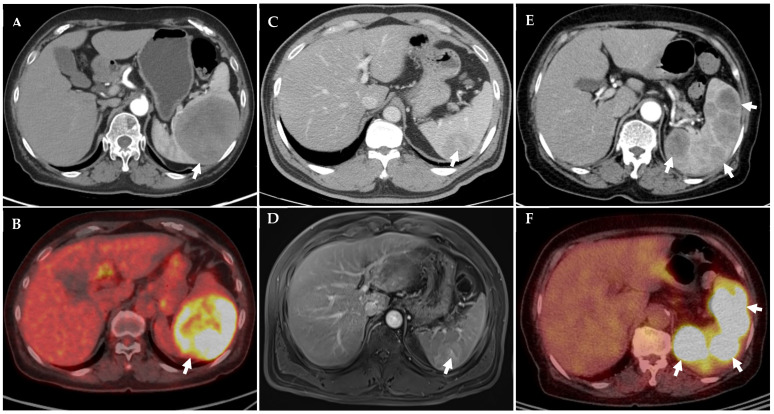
Multimodality imaging features of EBV-related primary splenic tumors. (A) Case 1, CT: well-circumscribed enhancing splenic mass measuring 8.5 × 7.5 cm (white arrow). (B) Case 1, PET/CT: FDG uptake greater than the liver, Deauville score 4 (white arrow). (C) Case 4, CT: enhancing splenic lesion with central stellate scar, measuring 5.2 × 4.0 cm (white arrow). (D) Case 4, MRI (delayed phase): heterogeneous prolonged enhancement with central scar (white arrow). (E) Case 5, CT: multiple hypodense splenic lesions, largest measuring 9.5 × 6.4 cm (white arrows). (F) Case 5, PET/CT: hypermetabolic activity confined to the spleen (white arrows).
